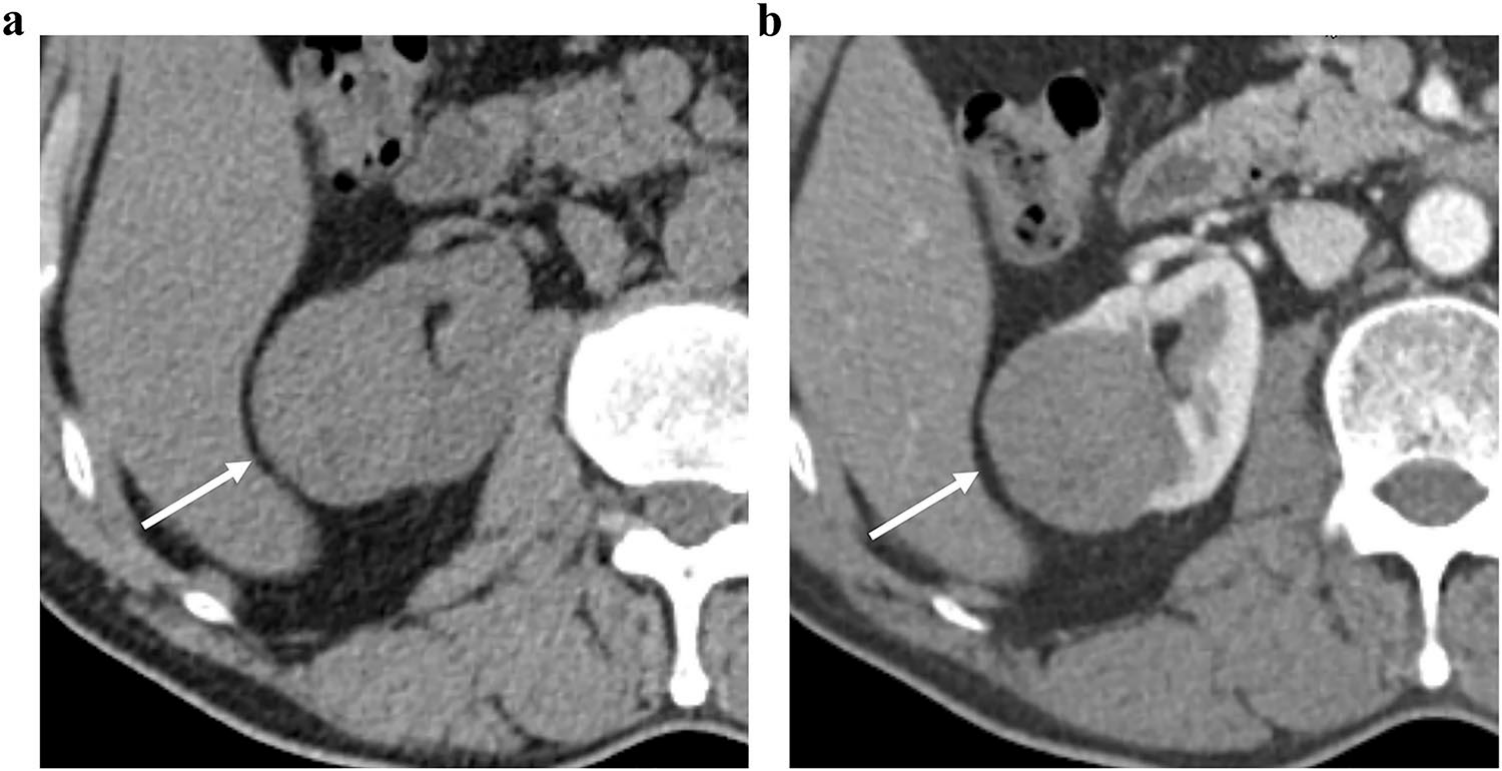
A 66-year-old man with type 1 papillary renal cell carcinoma. a The pre-contrast CT image showed a 43-mm tumor with low attenuation (35 HU) in the left kidney lower pole. b The tumor showed homogeneous enhancement in the corticomedullary phase
